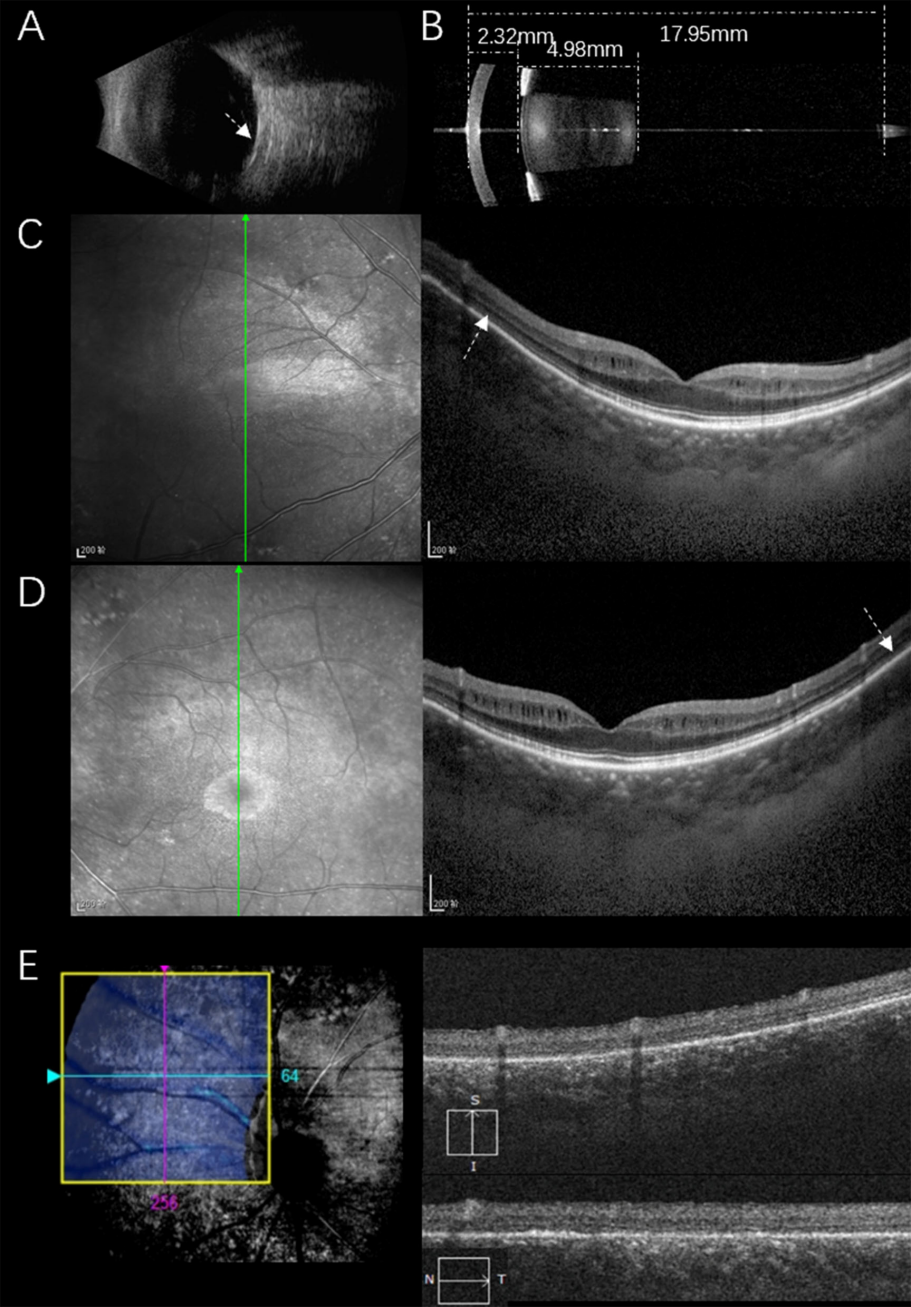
Ocular biometric measurement and OCT scan for the proband. B-mode ultrasonography showed a short eye axis, scleral thickening [white arrow in (A)] and IOL-Master results showed that the anterior chamber depth was 2.32 mm, the lens thickness was 4.98 mm, and the axial length was 17.95 mm (B). OCT scan showed diffuse macular thickening, splitting of the inner retinal layers with discrete bridging elements at the parafovea, and the outer retinal thickness was decreased and pointed by white arrows in (C,D), the reduced retinal thickness and the absent ellipsoid band were more apparent in the nasal retina around the optic disc indicating atrophy of the outer layer of the retina (E).
